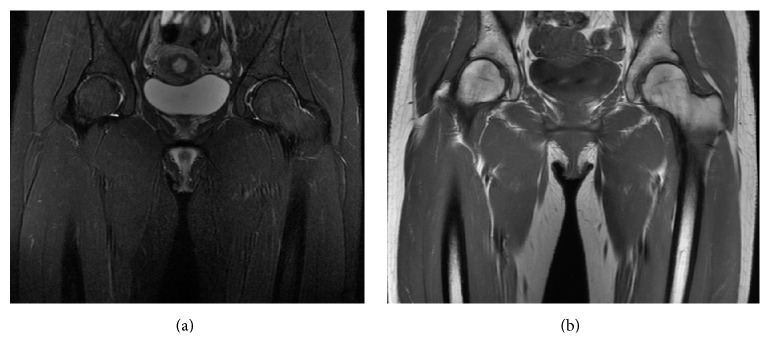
Magnetic resonance images of pelvis seven weeks after symptom onset. (a) Coronal T2-weighted magnetic resonance image. Normal signal uptake within the femoral heads and necks bilaterally. (b) Coronal T1-weighted magnetic resonance image. Normal signal uptake within the femoral heads and necks bilaterally.
